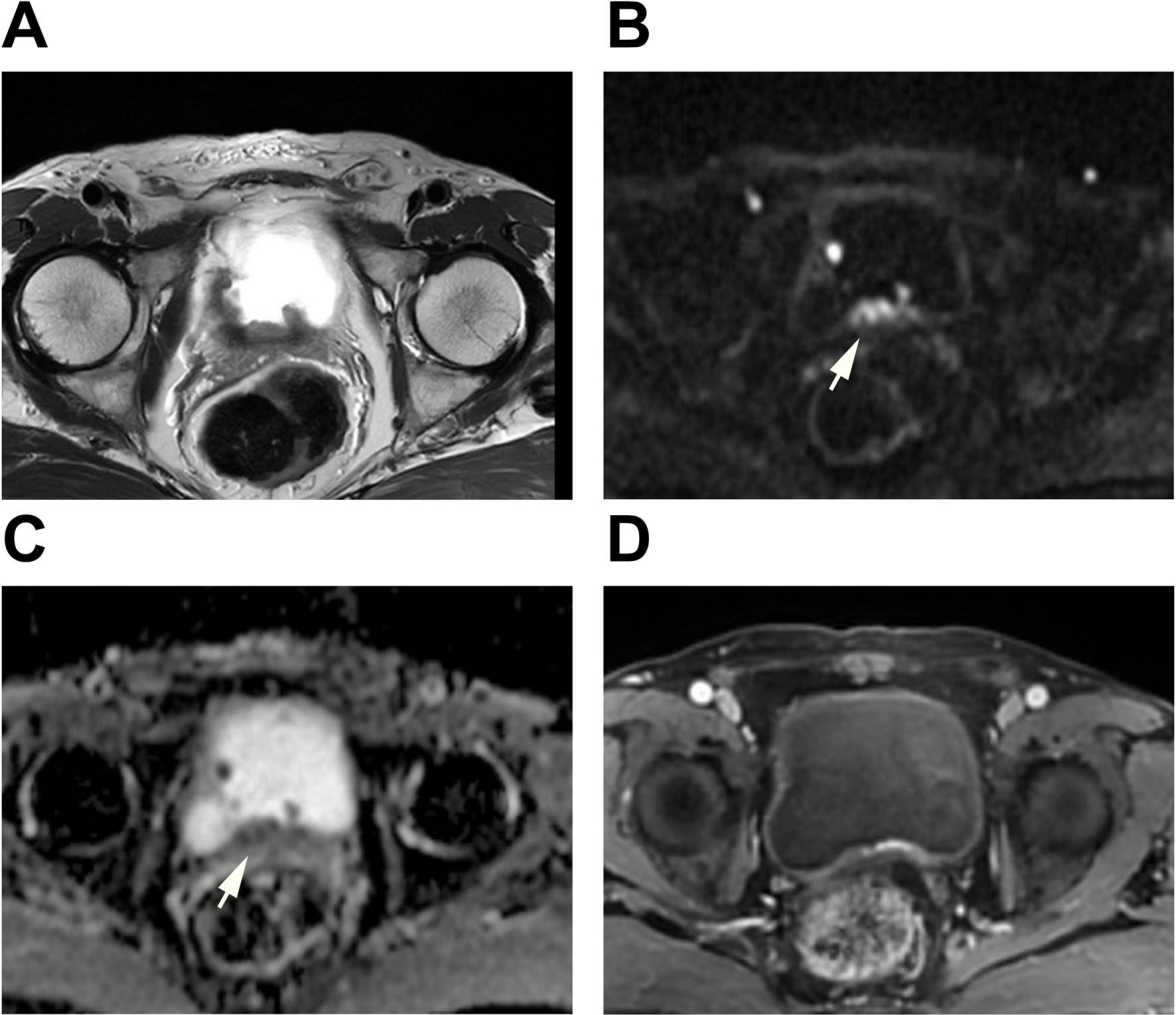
A case was evaluated using three evaluation methods. A patient over 65 years old with hematuria underwent mp-MRI prior to the initial TURBT. T2WI showed multiple sessile tumors on the trigone, dome, right wall, and posterior wall of bladder. The tumor located on the trigone, possessing the highest VI-RADS score, was selected for analysis. Clear stalks and thickened inner layers were observed on DWI and ADC map (Arrow). All readers assigned a T2WI score of 3 and a DWI score of 2, resulting in bp-DWI VI-RADS score was 2 and bp-T2WI VI-RADS score was 3. DCE did not show any clear disruption of muscularis propria. Therefore, all the readers assigned a DCE score of 3, resulting in a VI-RADS score of 3 for mp-MRI. T stage after TURBT was T2-high grade with glandular differentiation. The pathology results from the subsequent radical cystectomy confirmed this observation. A: T2WI; B: DWI; C: ADC; D: DCE
